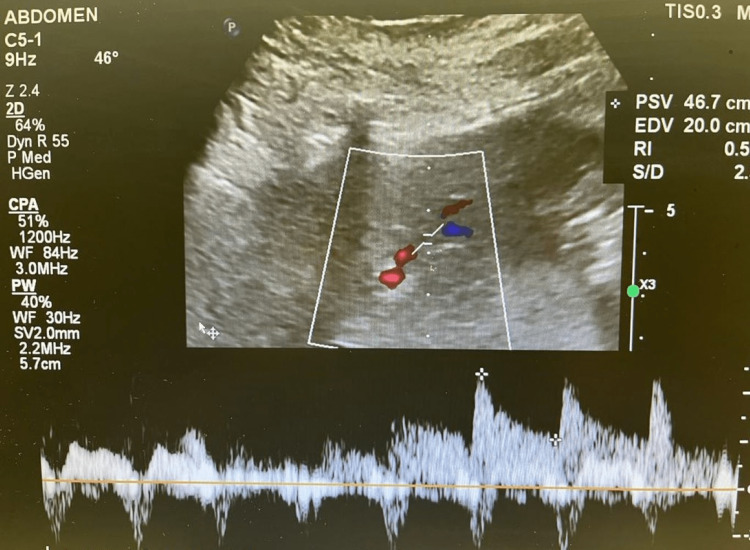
Doppler examination of the left hepatic artery on the first postoperative day
